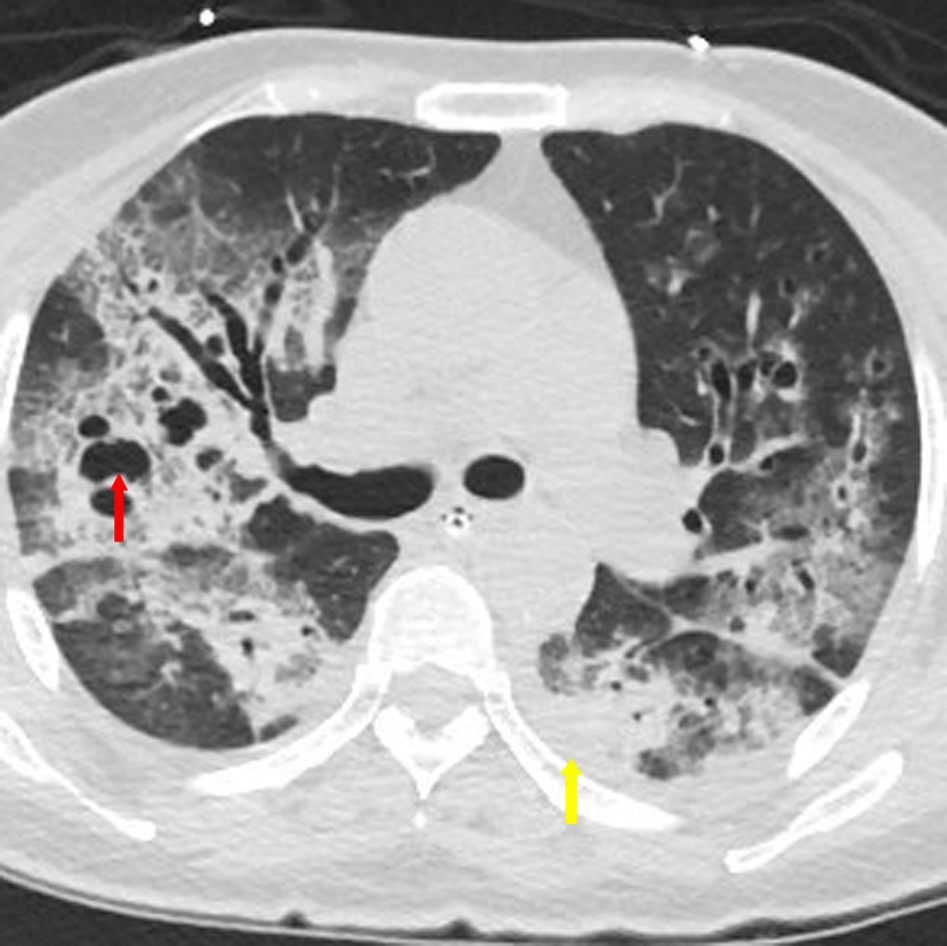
HRCT findings of a 65-year-old female case with staphylococcus aureus pneumonia. The red arrow showed the cavity, the yellow arrow showed the pleural effusion.
